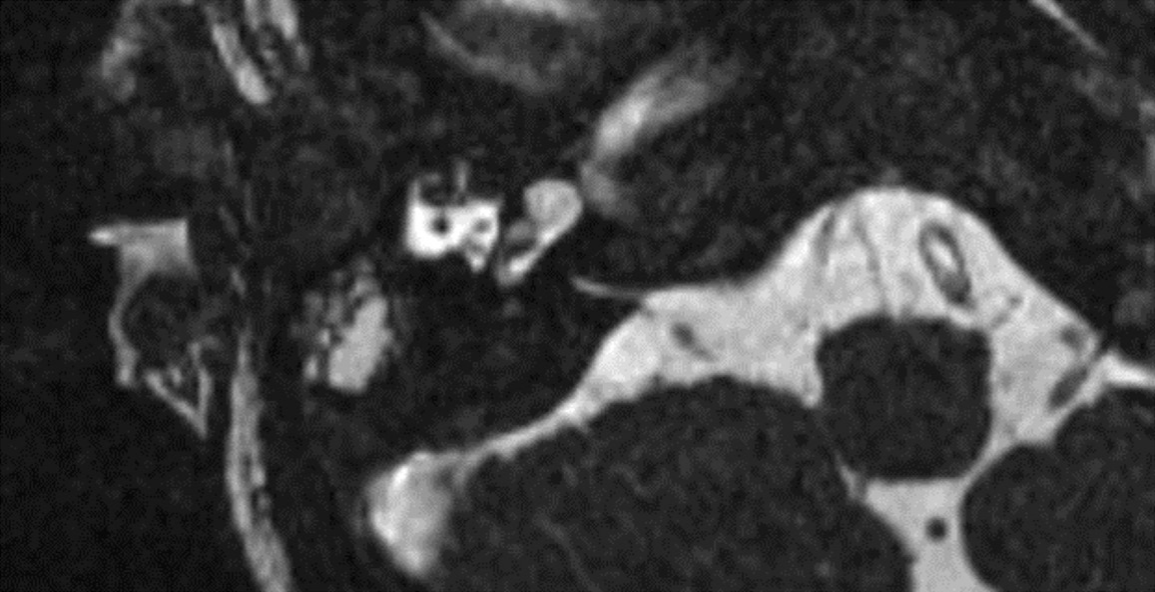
Postoperative CT scan of the cochlea showing the localization of the implant.
